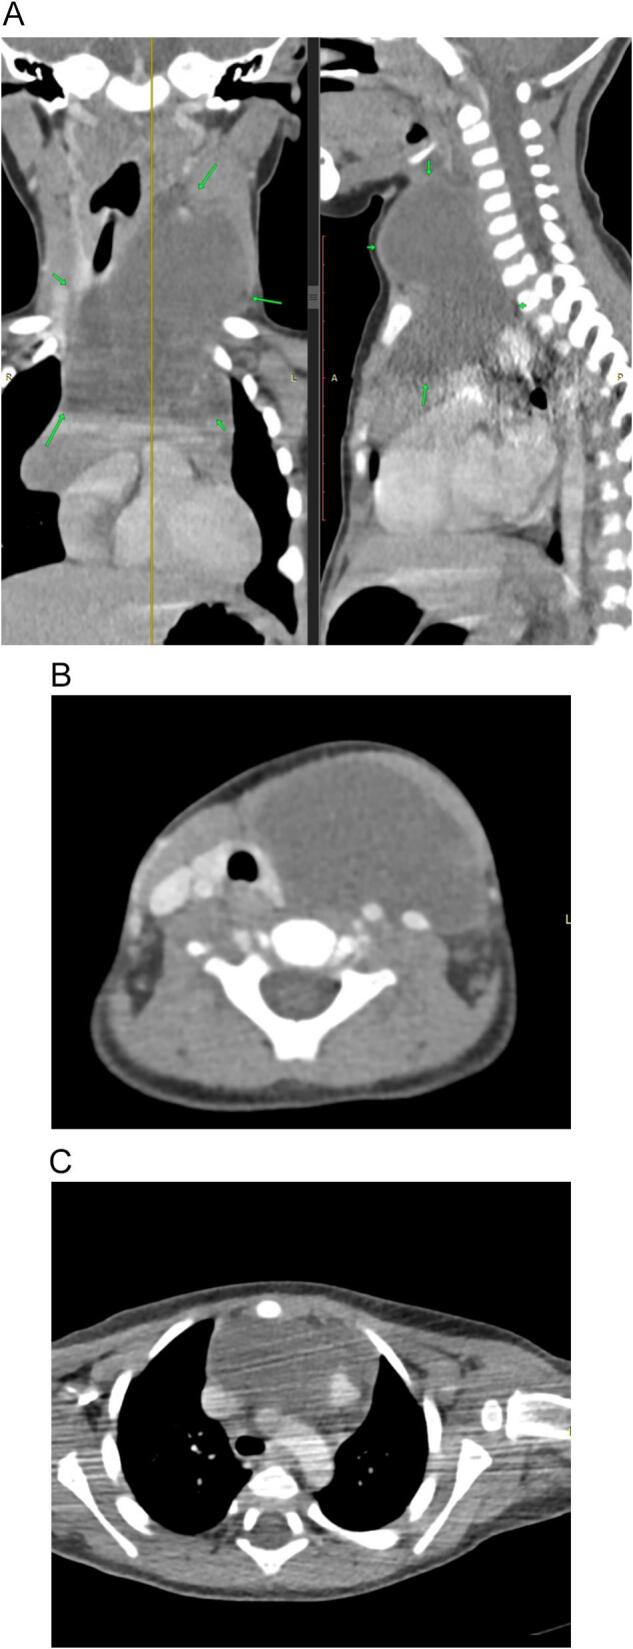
A: CT scan with contrast (sagittal and coronal planes) demonstrate well defined large cystic mass measures about (4 × 7 × 9 cm), with thin wall. The lesion extends from the left submandibular space inferiorly posterior to the sternocleidomastoid muscle, and retrosternally to the aortic arch level. B: CT scan with contrast (axial plane) demonstrates the lesion exerts moderate mass effect on the larynx causing a shift to the right and posterior shift to the left carotid sheath components. From the Posterior aspect, the mass reaches the prevertebral space. C: CT scan with contrast (axial plane) demonstrates retrosternally extension to the aortic arch level.
